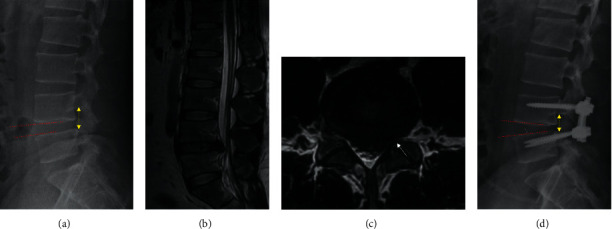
Images of the case: (a) preoperative lateral X-rays; (b) preoperative sagittal MRI; (c) preoperative axial MRI; and (d) postoperative lateral X-rays.
